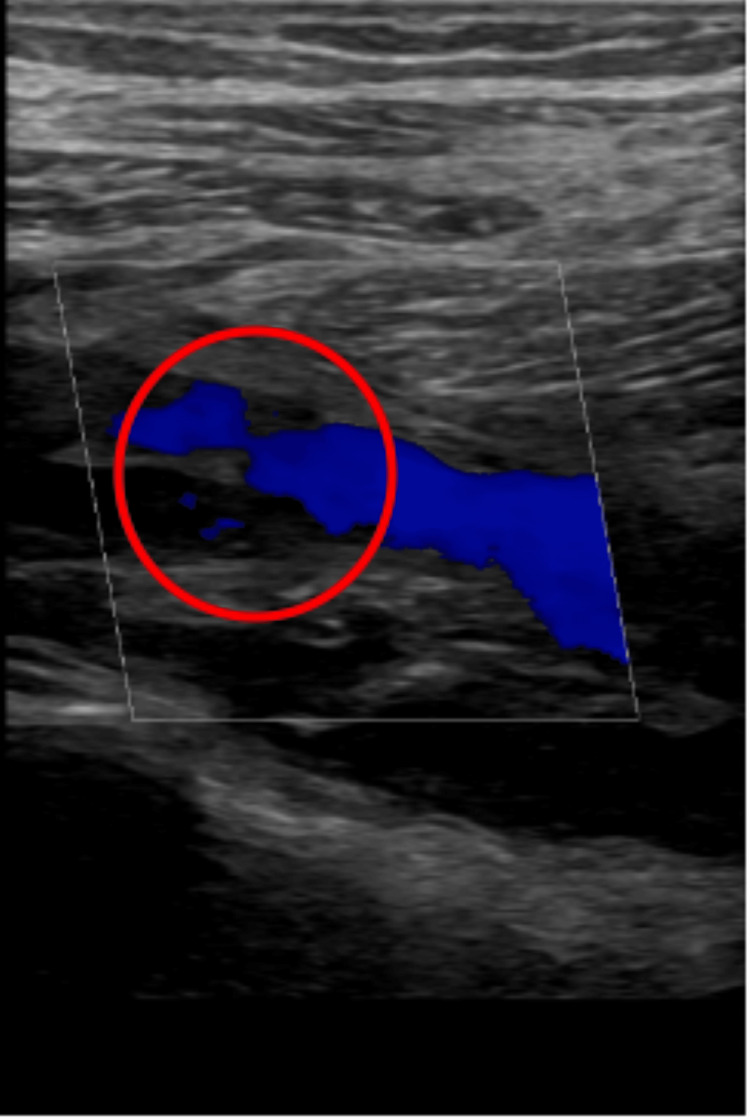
Ultrasound demonstrating occlusion of the left popliteal artery
